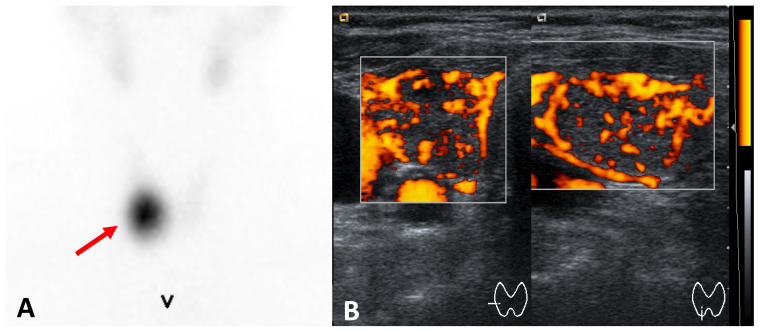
Toxic adenoma (right thyroid lobe) (A) ^99m^Tc-pertechnetate scintigraphy: hyperfunctioning nodule (arrow) and functional suppression of the remaining thyroid tissue; ultrasound ((A) axial, (B) longitudinal views): hypoechoic and well-demarkated nodule with increased vascularity.
